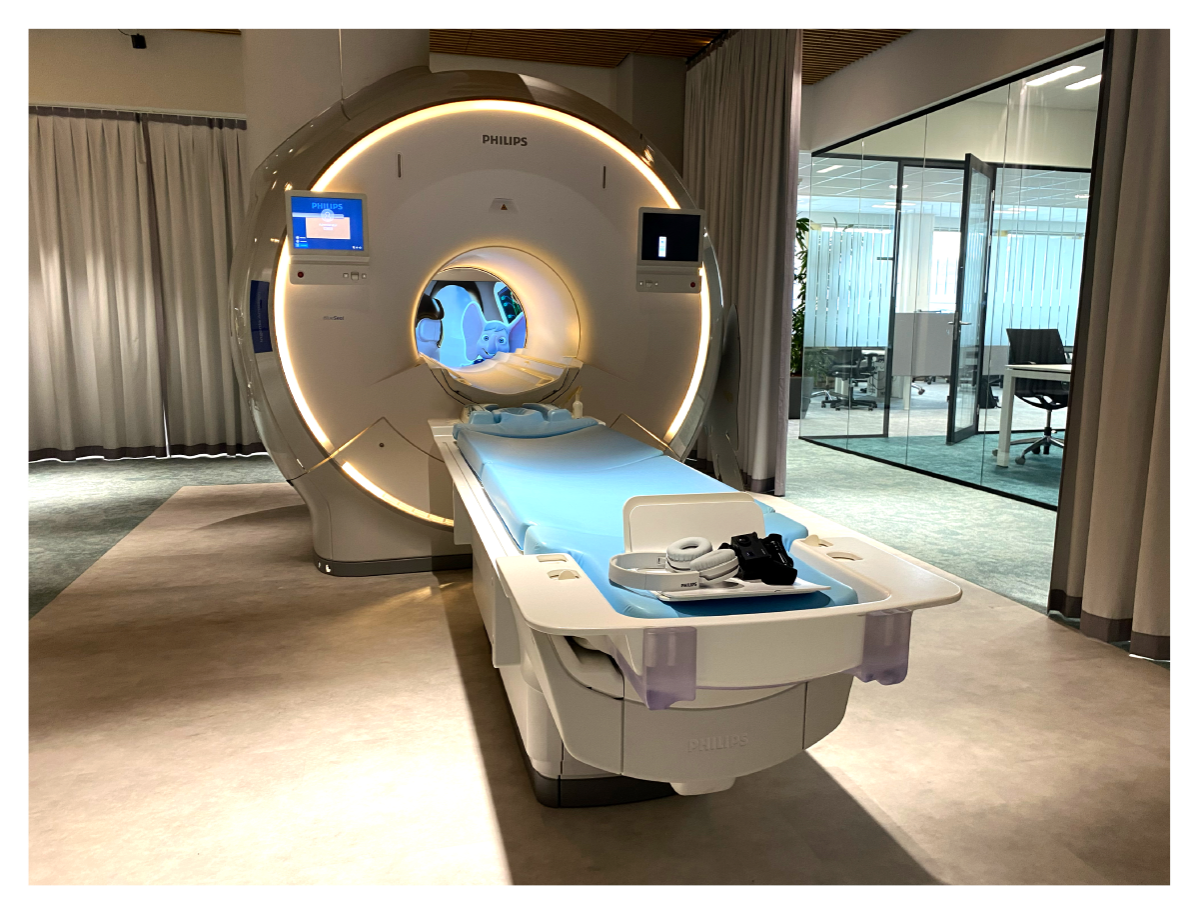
Picture of the mock MRI scanner in the usability laboratory, where the ~10-minute mock examination with the healthy participants (N=10; aged 5-10 years) of the pediatric In-bore usability study took place. MRI: magnetic resonance imaging.
